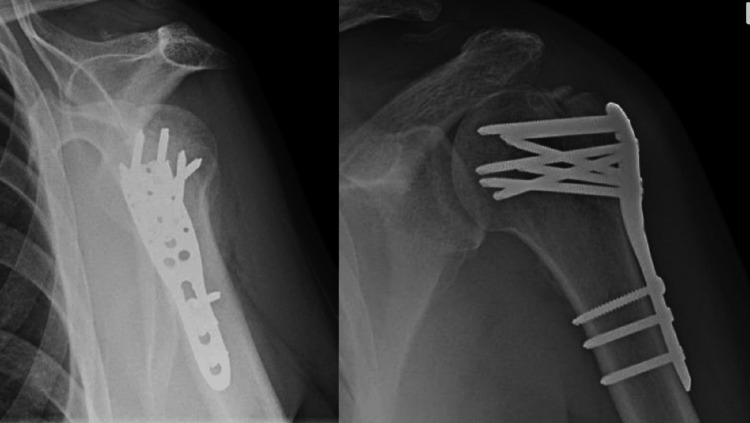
Post-operative antero-posterior and lateral radiographs These demonstrate anatomical reduction of the tuberosity and an advanced degree of union of this fragment.
